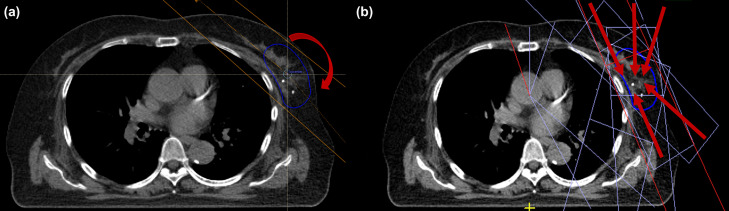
Examples of the (a) VMAT and (b) S&S IMRT plan setup and beam layout on the planning CT image. The number of red arrows indicates the number of beams, and the red arrow direction indicates beam direction (Blue: PTV).
